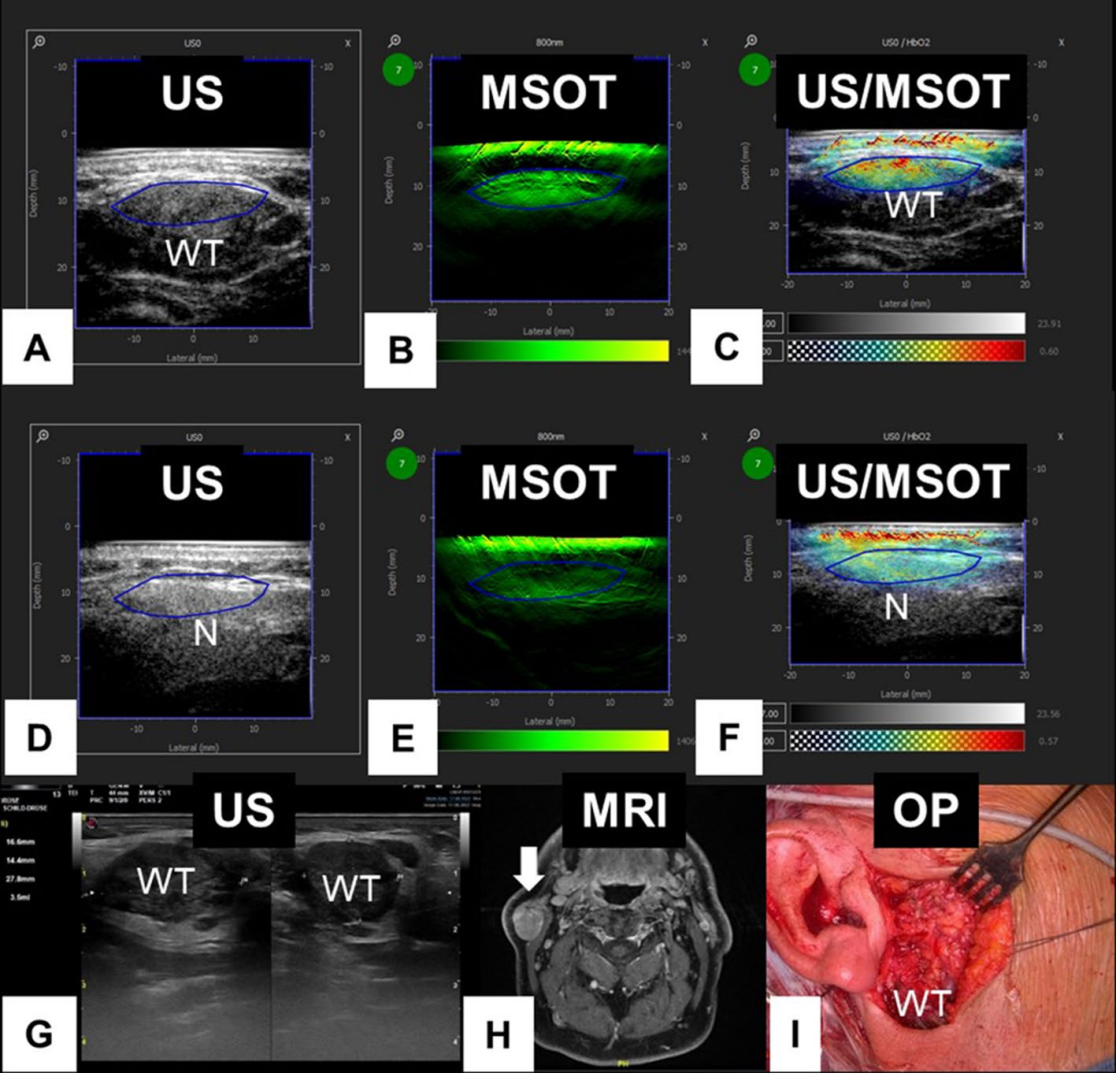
Example of a Warthin tumor (WT) of the right parotid gland. (A–C) Parotid gland with tumor; (D–F) contralateral healthy parotid gland. (A) Ultrasound (US) image of the MSOT system; part of WT circled in blue. (B) MSOT image of 800 nm; Region of interest (ROI) circled in blue. (C) Hybrid US/MSOT image; ROI circled in blue. (D) US image of the MSOT system; ROI in normal (N) salivary gland tissue circled in blue. (E) MSOT image of 800 nm; ROI in normal tissue circled in blue. (F) Hybrid US/MSOT image; ROI in normal tissue circled in blue. (G) High-resolution image with normal US device. (H) Magnetic resonance imaging (MRI) showing the tumor (arrow) in the parotid gland. (I) Intraoperative (OP) situs. The tumor does is covered by normal parotid tissue and does not only partly reach the surface of the parotid gland.
